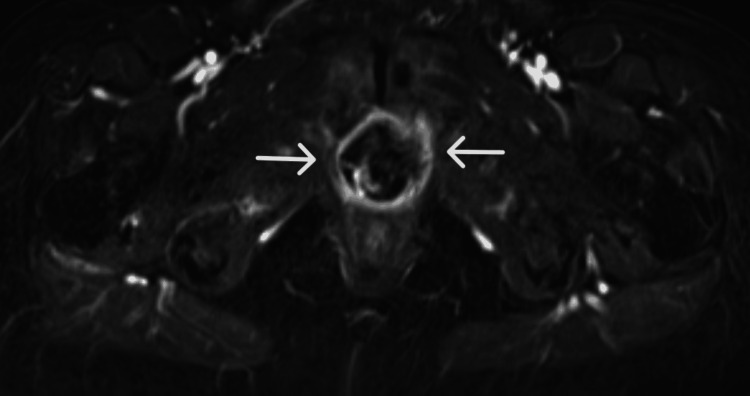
Axial T1-weighted postcontrast subtraction magnetic resonance image of a periurethral mass demonstrating peripheral and solid nodular central enhancement, denoted by the white arrows
